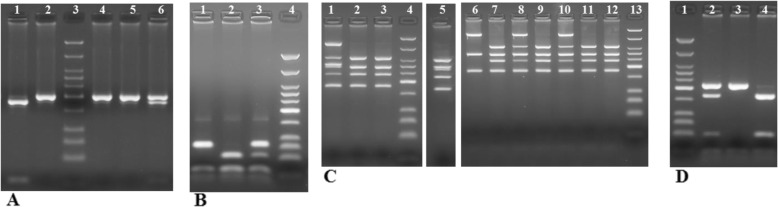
Electrophoretic separation of *MTHFR*, *XRCC1* and *OGG1* digestion in 3% Metaphor gel. Marker of molecular weight: Low Molecular Weight DNA Ladder (NEB). a*MTHFR* C677T: lane 1 TT (178, 23 bp), lanes 2, 4, 6 CC (201 bp), lane 6 CT (201, 178, 23 bp), lane 3 marker molecular weight; b*MTHFR* A1298C: lane 1 CC (84, 31, 30, 28, 18 bp), lanes 2 AA (56, 31, 30, 28, 18 bp), lane 3 AC (84, 56, 31, 30, 28, 18 bp), lane 4 marker molecular weight; c*XRCC1* Arg194Trp (bold) and Arg399Gln PCR-multiplex: lane 1 arg/trp-arg/gln (615, 374, 313, 292, 241, 174 bp), lanes 2, 3, 7, 9, 11, 12 arg/arg-arg/arg (374, 292, 241, 174 bp), lane 5 arg/trp-arg/arg (374, 313, 292, 241, 174 bp), lane 6 arg/arg-gln/gln (615, 292, 174 bp), lanes 8,10 arg/arg-arg/gln (615, 374, 292, 241, 174 bp), lanes 4, 13 marker molecular weight. d. *OGG1* Ser326Cys: lane 1 marker molecular weight, lane 2 ser/cys (213, 164, 49, 21 bp), lane 3 ser/ser (213, 21 bp), lane 4 cys/cys (164, 49, 21 bp)
